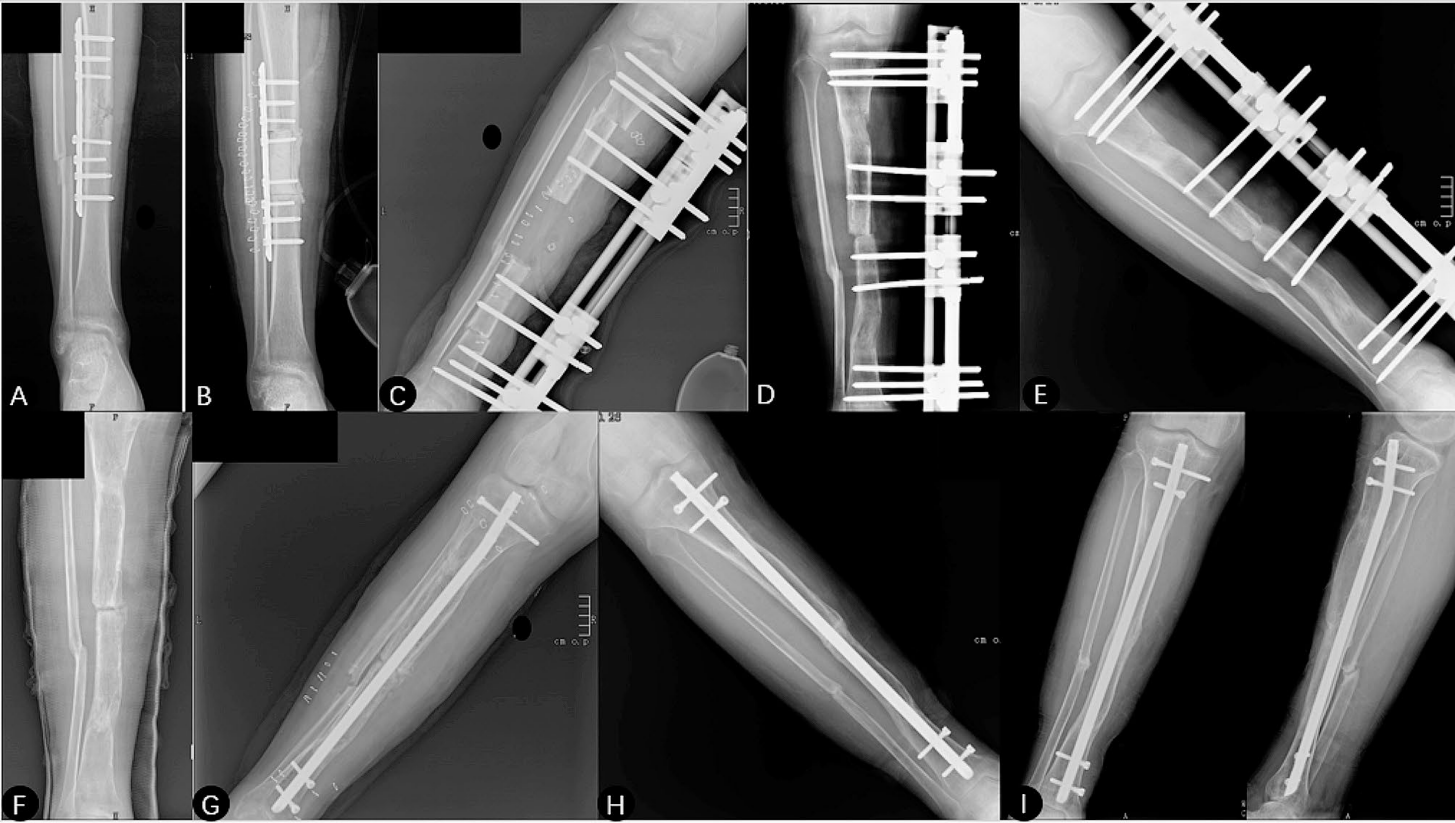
A 32-year-old male presented with fracture-related infection of the right tibia, which developed into chronic osteomyelitis, and underwent Trifocal bone transport using the Ilizarov technique. Docking site refracture was encountered 5.6 months after the removal of the external fixator, which was managed by Intramedullary nailing along with autologous bone grafting. (A) Anteroposterior radiograph showed nonunion and fracture-related infection after open reduction and internal fixation. (B) Anteroposterior radiograph showed chronic osteomyelitis after nonunion was managed by autologous bone grafting. (C) Anteroposterior radiograph on the first postoperative day after application of trifocal bone transport using the Ilizarov technique. (D) Anteroposterior radiograph during bone transport. (E) Anteroposterior radiograph before removal of external fixation after consolidated docking union. (F) The docking site refracture was encountered 6.6 months after the removal of the fixator and was managed temporarily by casting. (G) Anteroposterior radiograph demonstrated docking site refracture managed by intramedullary nailing. (H) Anteroposterior radiographs at 3-month follow-up. (I) Anteroposterior radiograph at 6.6 month follow-up time showed union at the docking site
